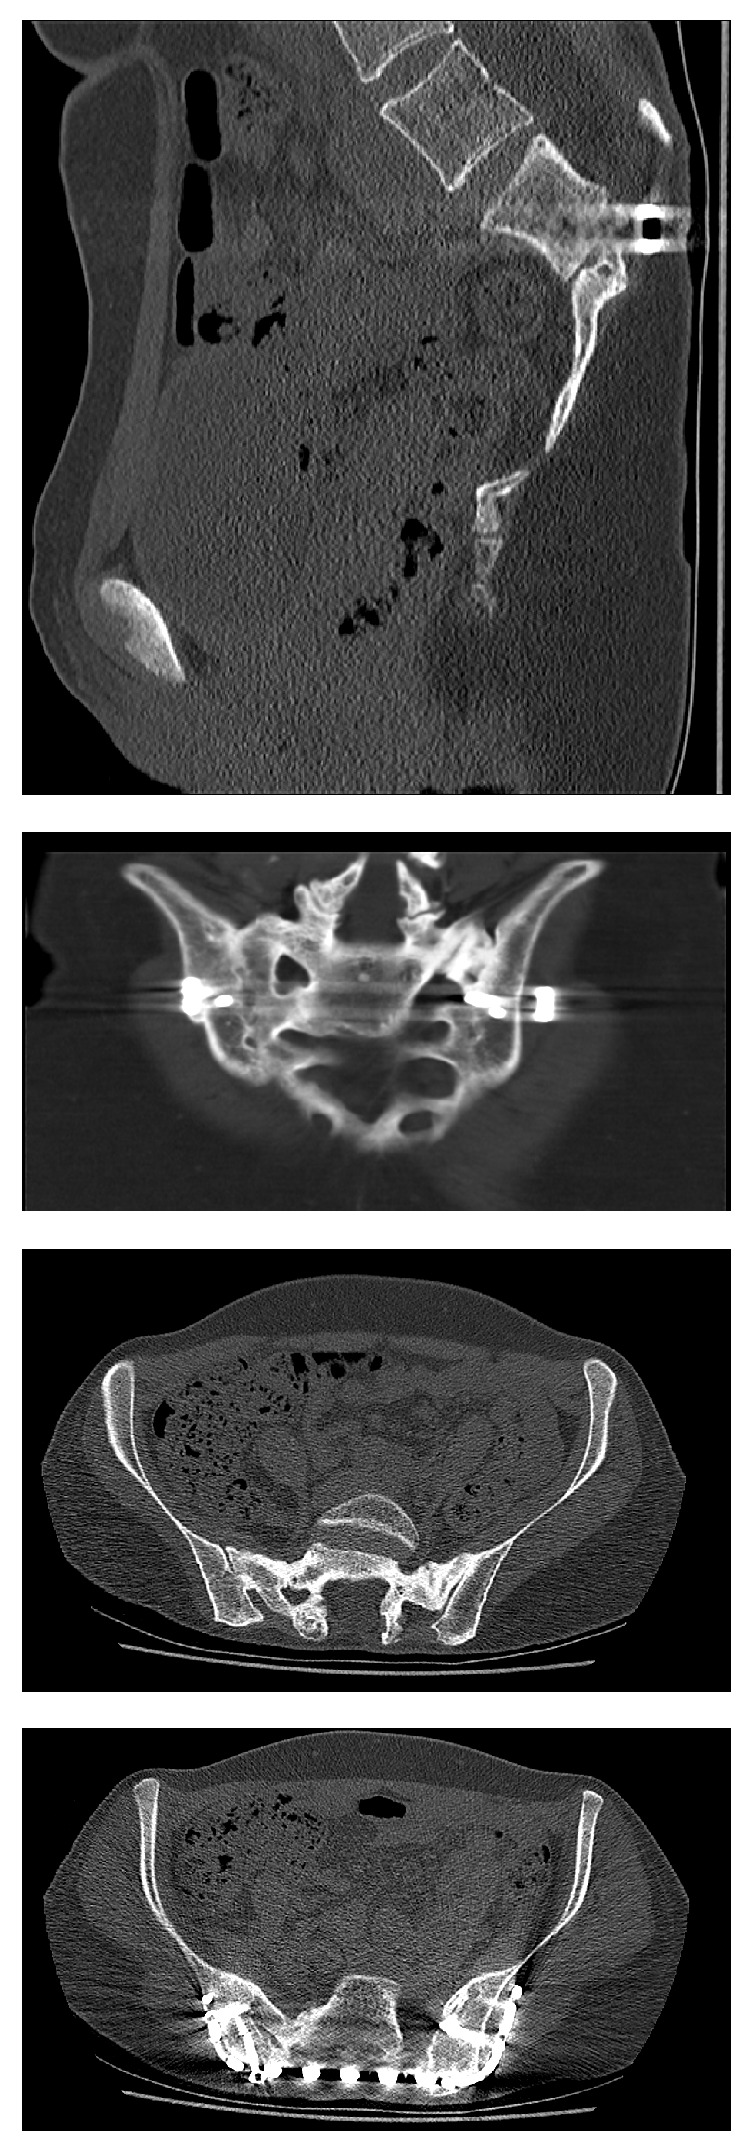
Selection of CT slices of the pelvis (CT and X-ray of the lower spine and pelvis were done in another hospital in 2015 due to lower back pain).
